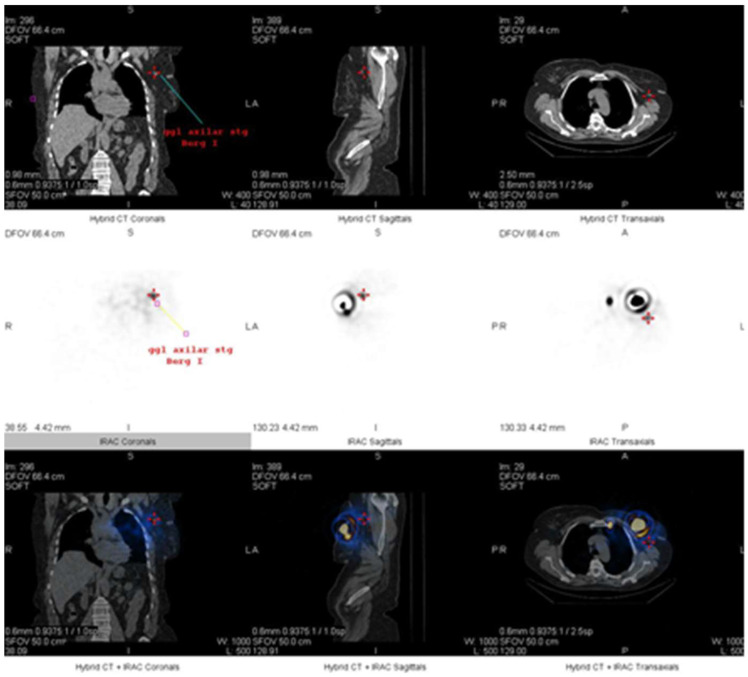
View at SPECT-CT of the SLN in the left axillary region: coronal, sagittal, and transverse images (CT, nuclear medicine, and fused series of images).
